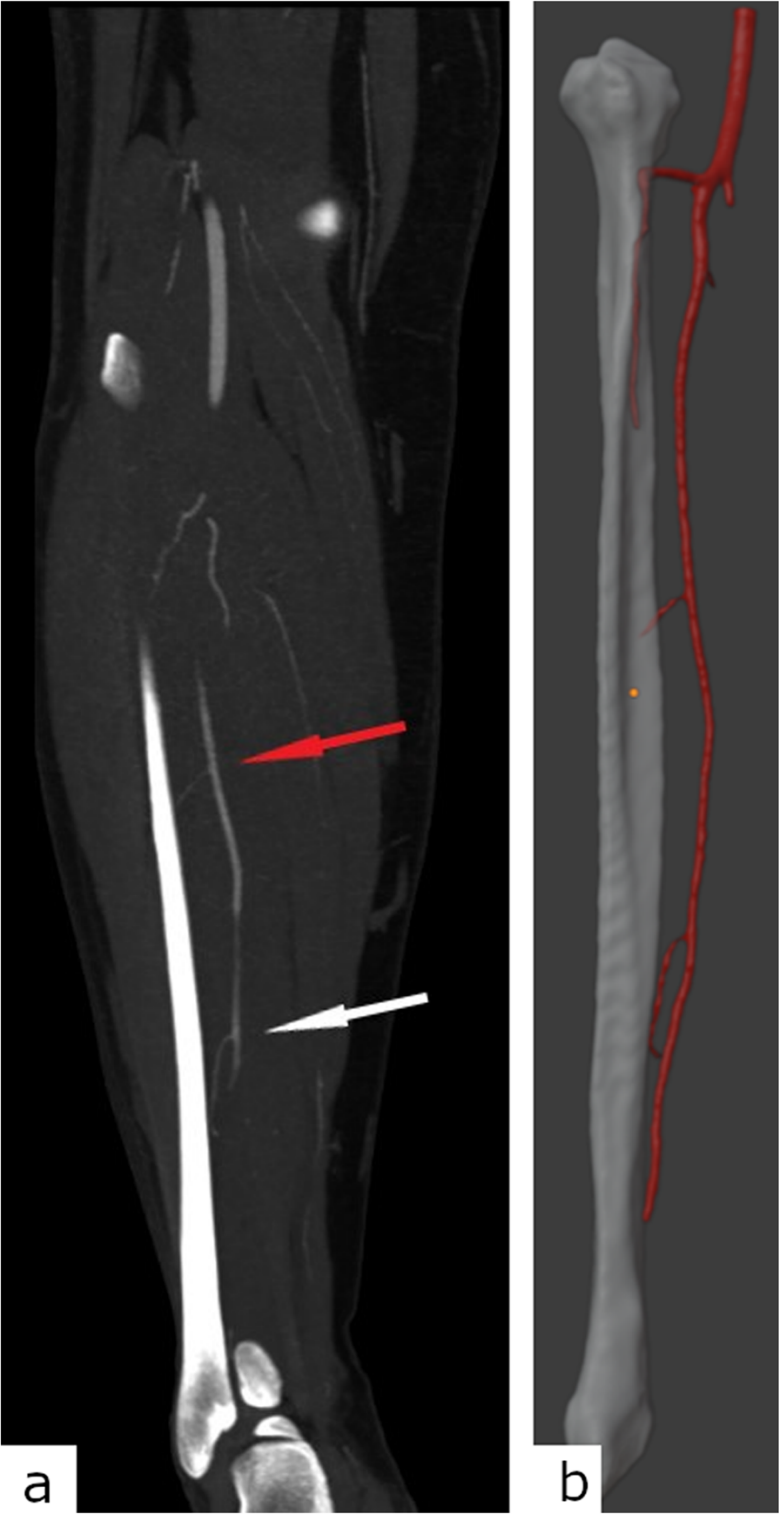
Visualisation of the peroneal artery perforators for the appropriate fibula orientation in space. a Coronal maximum intensity projection thick reconstructions of the right lower leg computed tomography angiography shows a good delineation of the proximal (red arrow) and distal (white arrow) peroneal artery perforators. b Matching three-dimensional model of the same patient’s right fibula and peroneal artery with visible perforators
